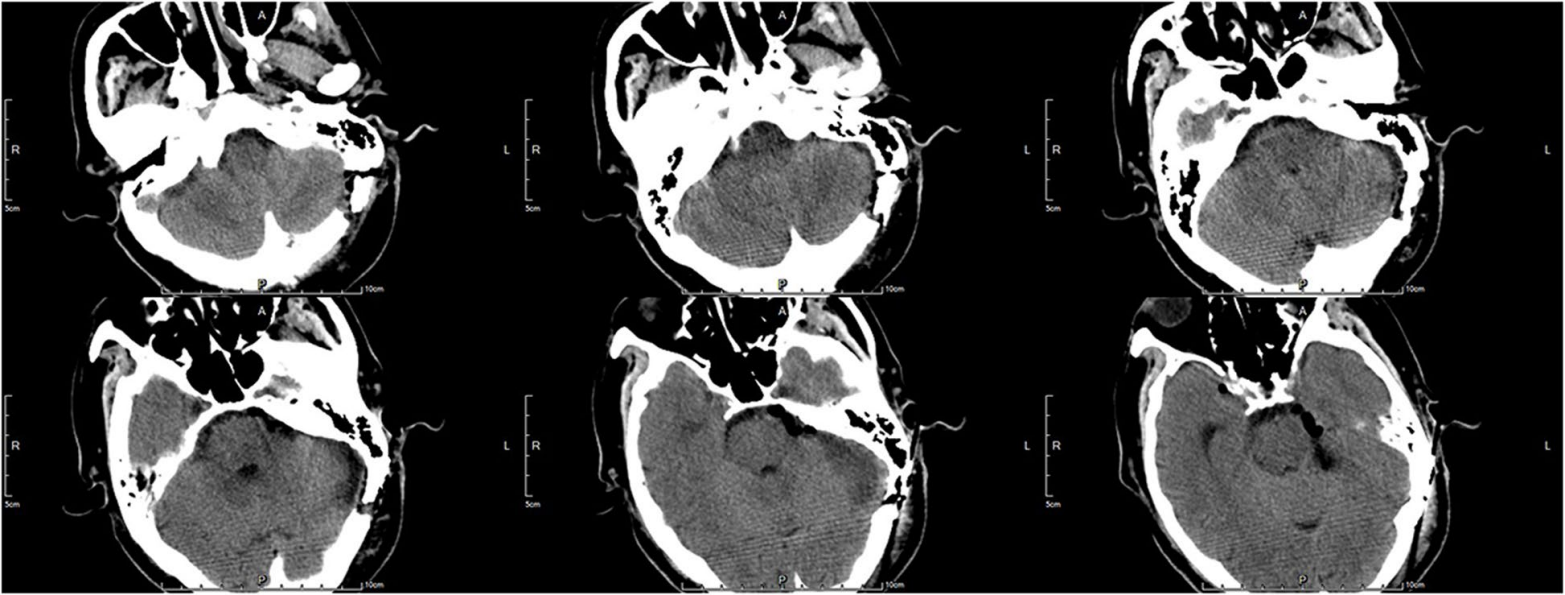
Postoperative CT images of case 1. The images showed the surgical area was clean
